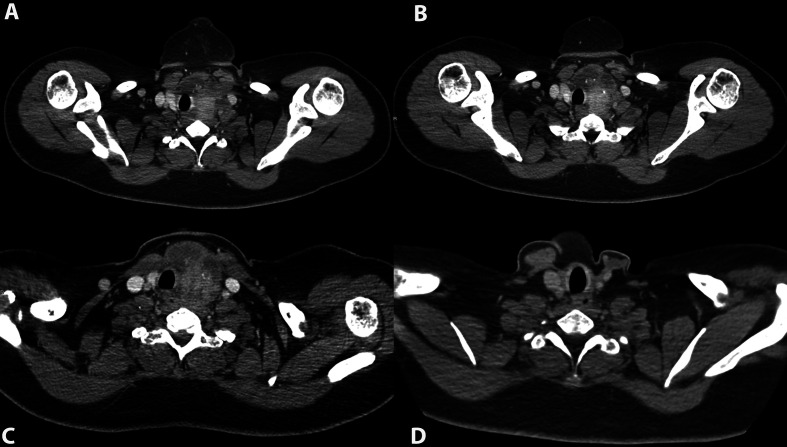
(A) Contrast computed tomography (CT) where a primary neoplasm of the left thyroid lobe and isthmus extends laterally to the common carotid artery with >180° encasement of same vessel and extension to prevertebral fascia, where no cleavage plane is observed. This lesion is associated to lymph node metastases in level III which encases partially the internal jugular vein (IJV) and produces major compression of the esophagus. (B) Lower axial view of the aforementioned neoplasm where extent to the mediastinum is demonstrated as well. Encasement of the left carotid artery, and major compression of the esophagus are demonstrated. (C) Contrast CT after EBRT (external beam radiotherapy treatment) where decrease in size of the aforementioned lesion located in the left thyroid lobe is observed. At this time, no major encasement of the carotid artery exists and a cleavage plane with common carotid artery and prevertebral fascia is demonstrated. Increase in soft tissue density is related to previous radiotherapy. The esophagus and IJV are non-compressed by the malignancy. (D) Contrast CT after a total thyroidectomy, left lateral, and central neck dissection. No suspicious lesions are observed, trachea and esophagus have no morphology alterations.
